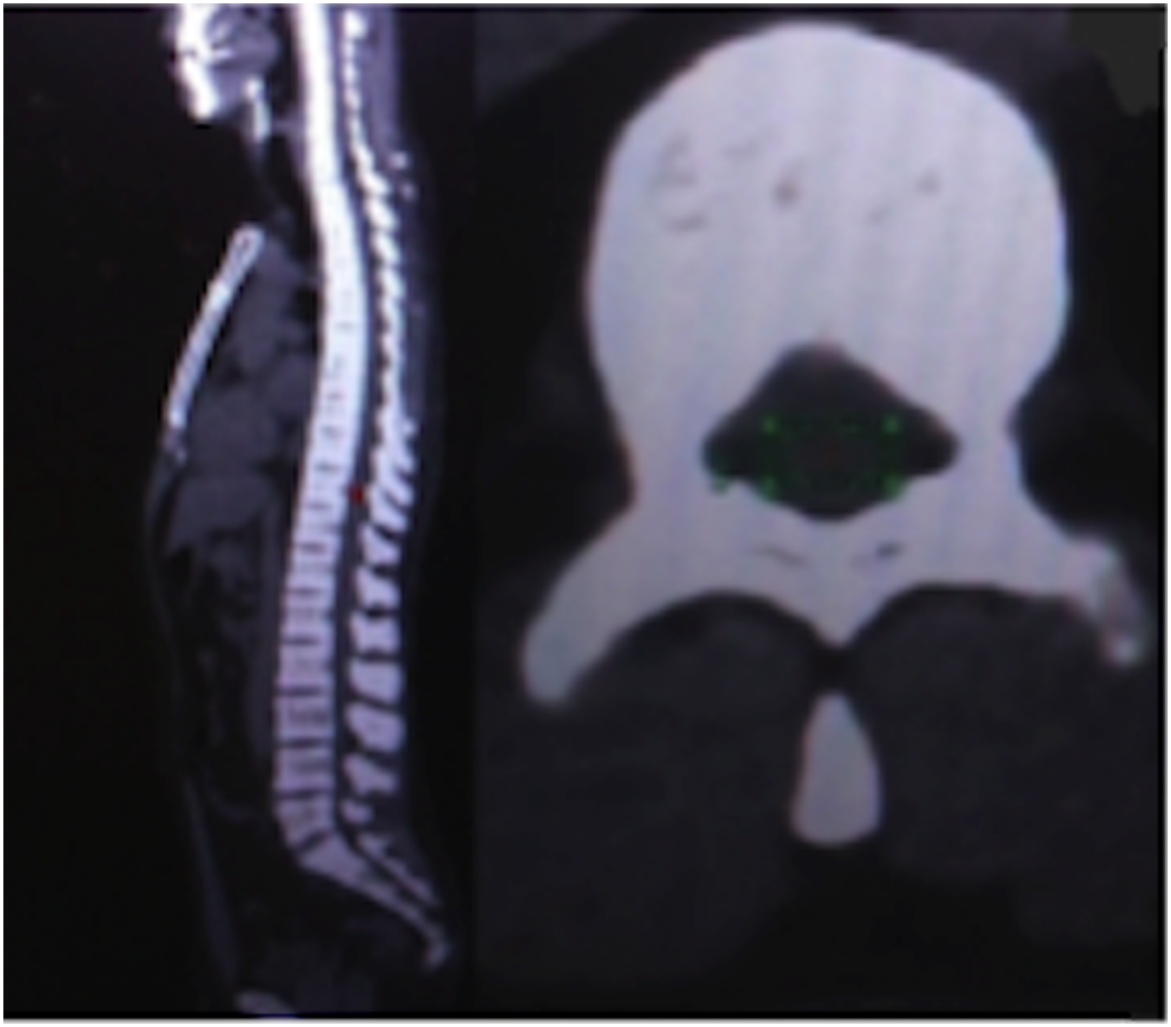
Sagital (left) and axial (right) PET/TC image. Area of measurement of the SUV, plane axial (green).
